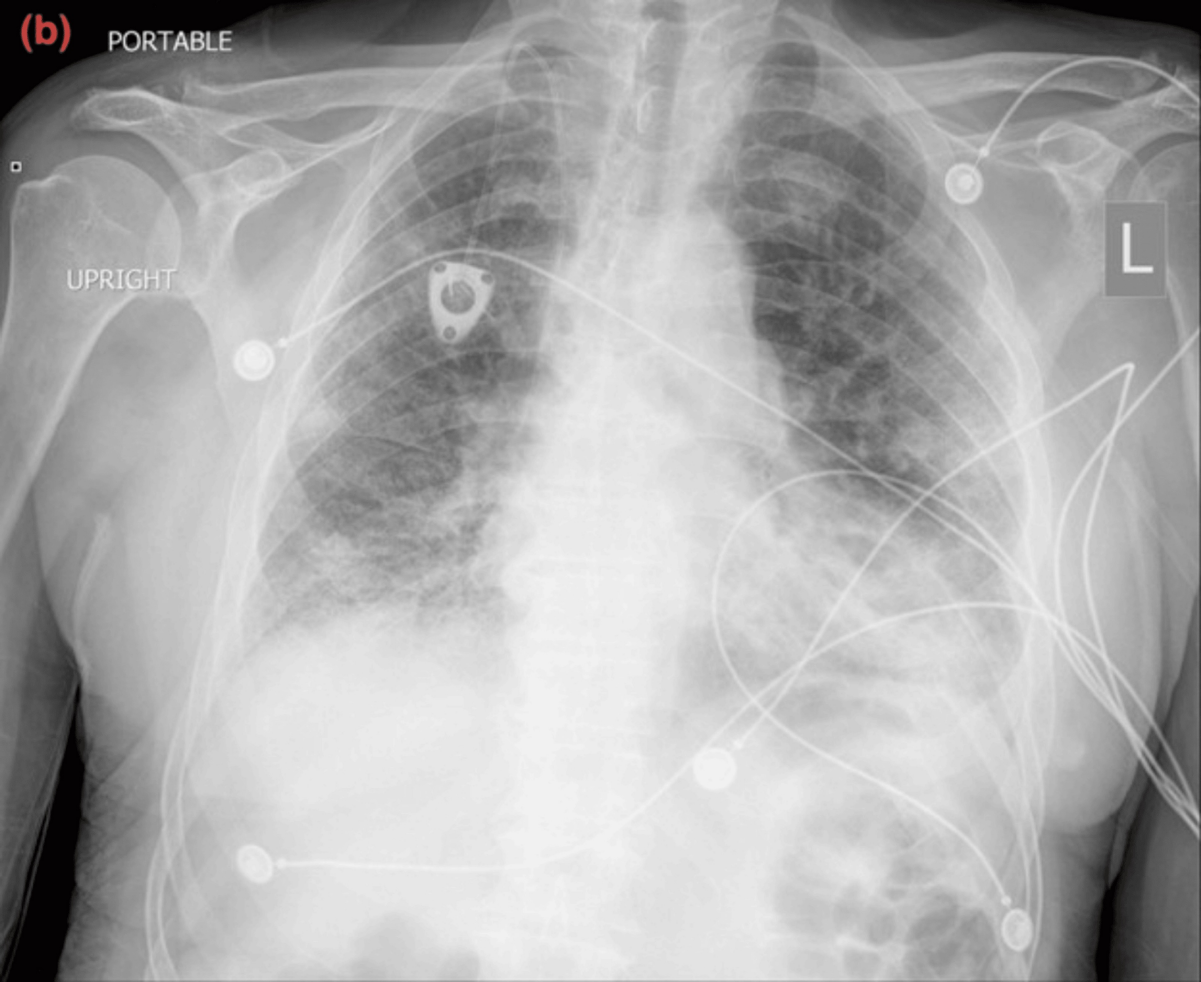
Progression of the disease on chest X-ray: (b) Chest X-ray on day of admission showing bilateral interstitial markings with patchy infiltrates.
